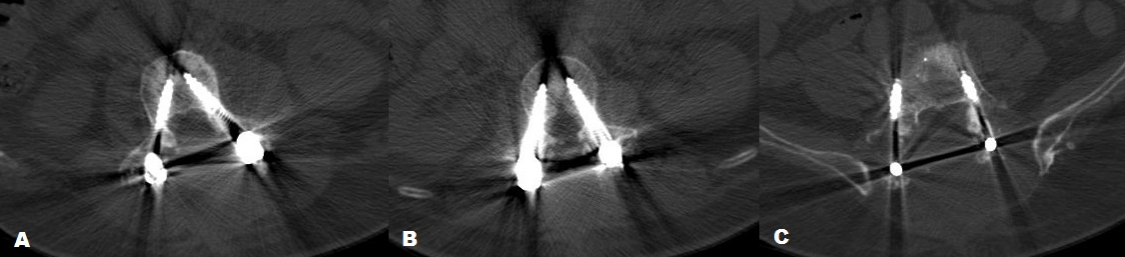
Computed tomography (CT) scan of fluoroscopy-guided hand-placed screws (A) demonstrates Grade-A accuracy for screw placement at L3 level (B) demonstrates Grade-B accuracy at L2 level. (C) Right screw demonstrates Grade-C placement and left shows Grade-B placements at level L5 according to Gertzbein-Robbins classification.
